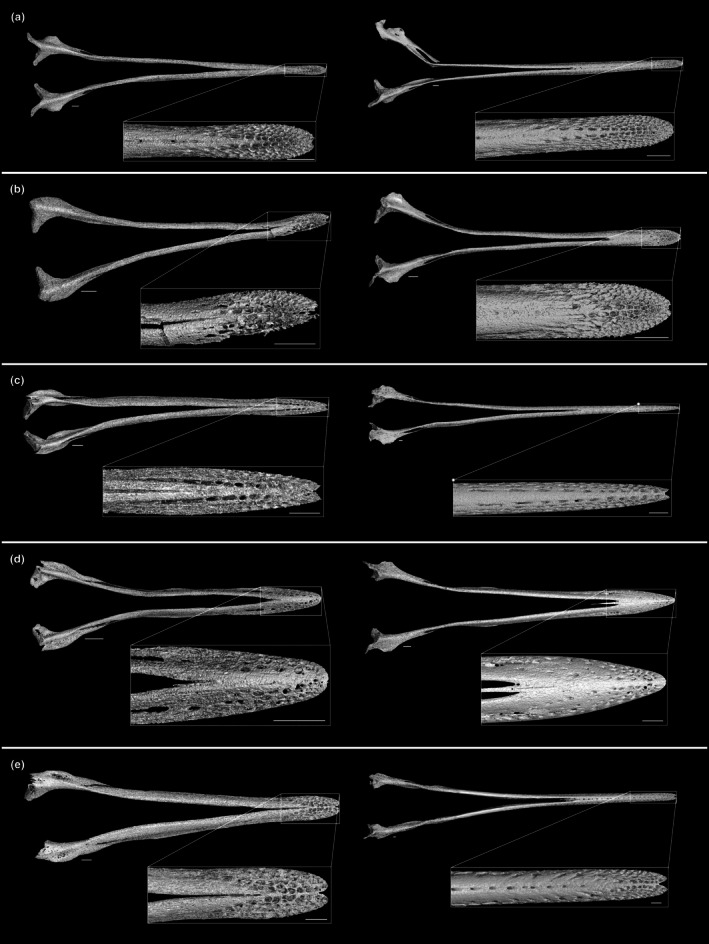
The mandibles of five species of scolopacid shorebirds in ventral view taken from CT scans (juveniles on left, adults on right), with insets showing the tip of the bill to highlight the numerous neurovascular pits in the bone. (a) *Calidris ferruginea*; (b) *C. minuta*; (c) *Tringa erythropus*; (d) *Phalaropus fulicarius*; (e) *Numenius phaeopus*. Scale bars = 1 mm.
